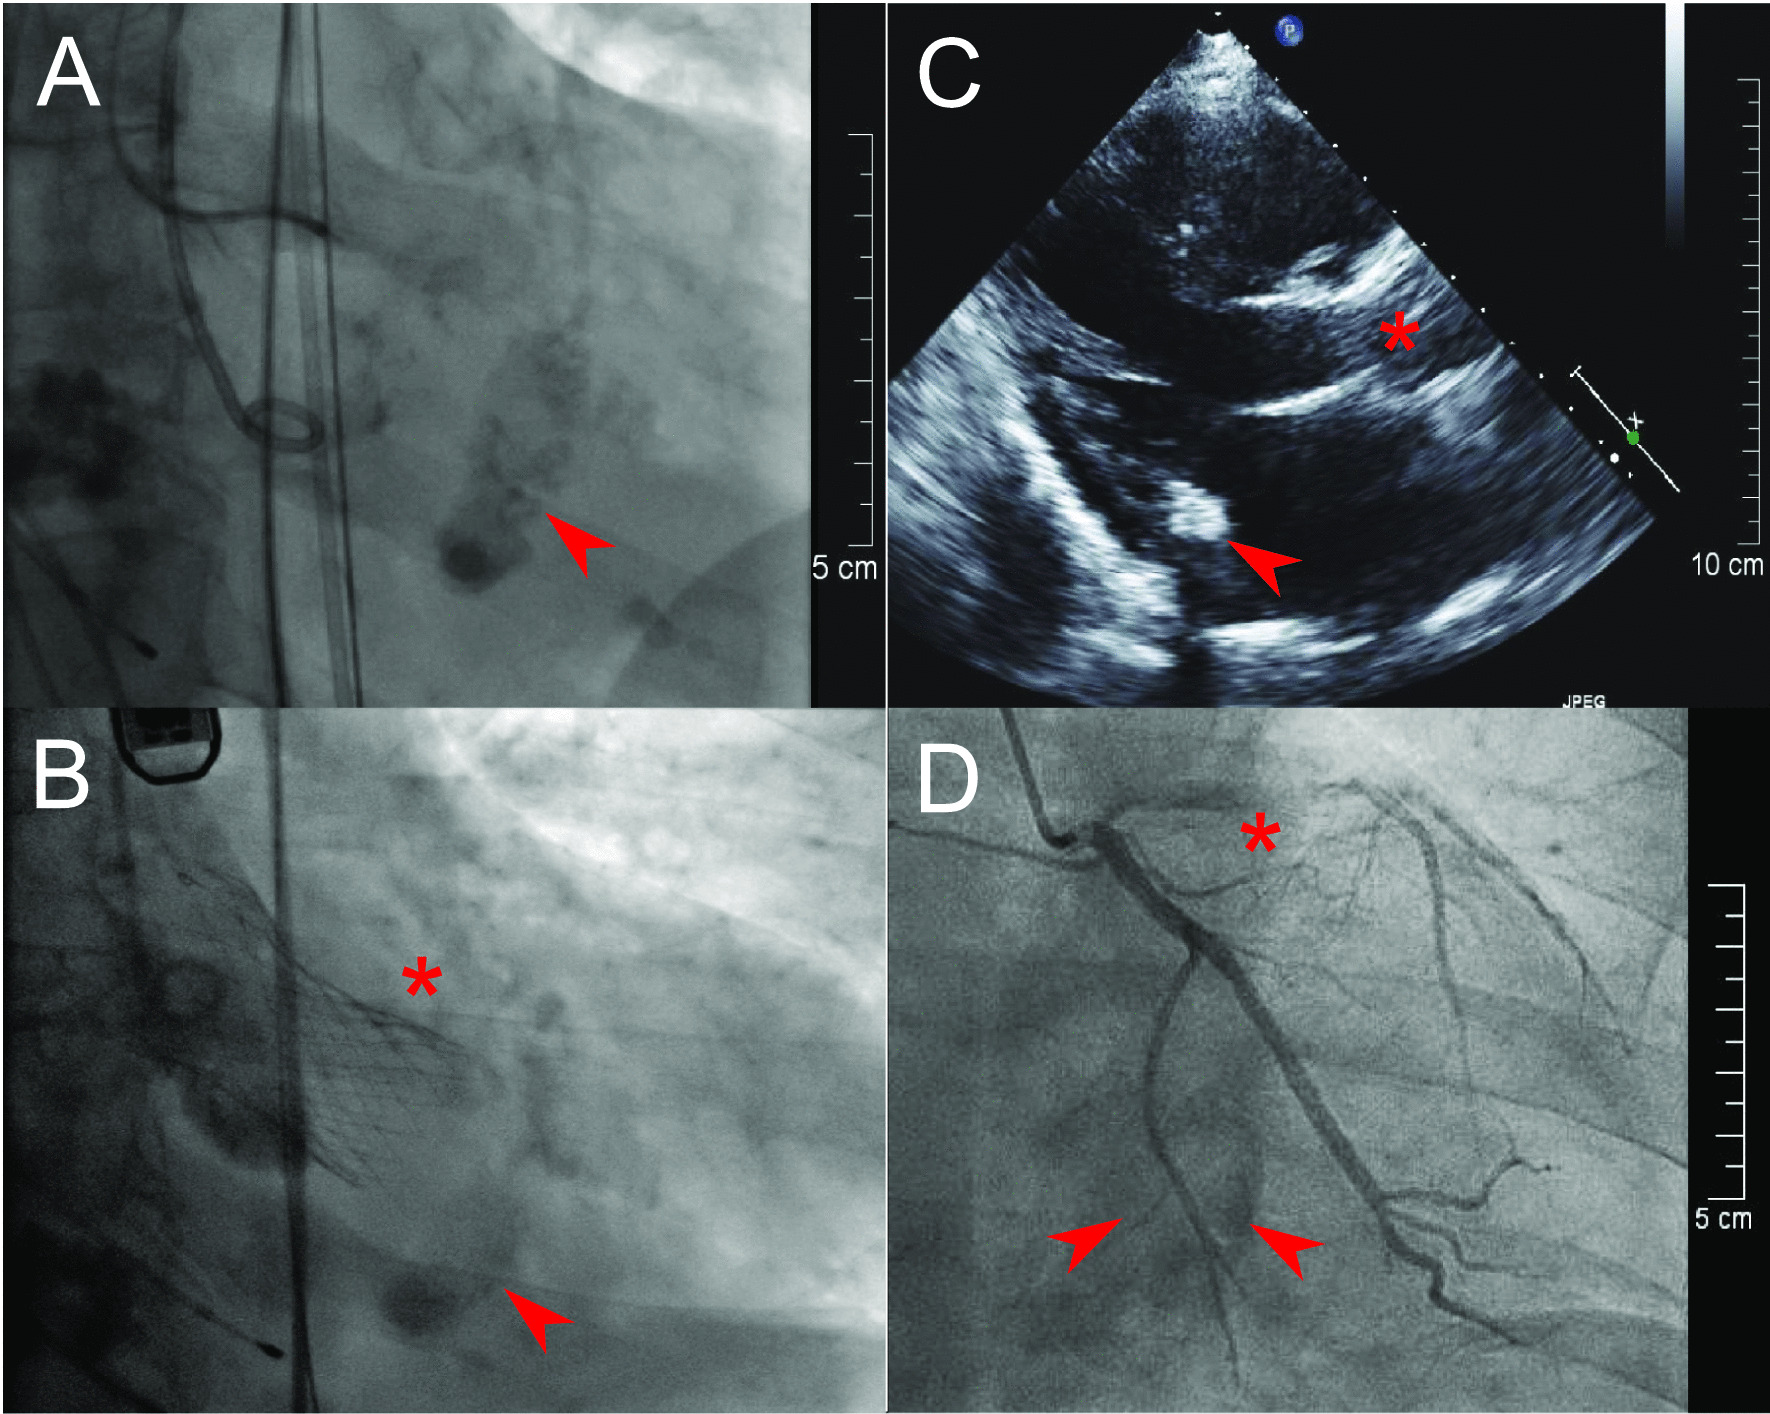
Before (A) and after (B) transcatheter aortic valve prothesis (star) for severe aortic stenosis with CMAC (arrow) at the inferior mitral valve annulus in case 4. Echocardiographic 1 year follow-up visit after transcatheter aortic valve replacement of case 1 (C) with the aortic prosthesis (star) and CMAC mass at posterior mitral valve annulus (star). Percutaneous coronary intervention of the left anterior descending artery (star) and the “shadow” of CMAC (arrow) at the bottom image of case 3 (D). *CMAC* caseous mitral annular calcification
